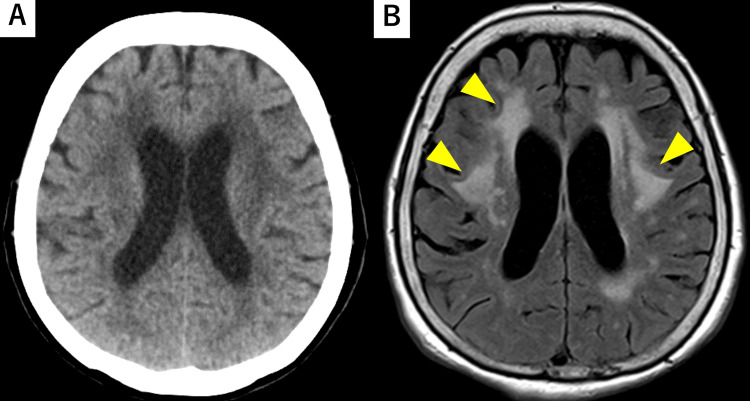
Head CT and MRI scan on admission Head CT (A) and FLAIR MRI (B) showing high signal intensity in the periventricular body of the lateral ventricle (yellow arrowheads). CT: computed tomography, FLAIR: fluid-attenuated inversion recovery, MRI: magnetic resonance imaging
